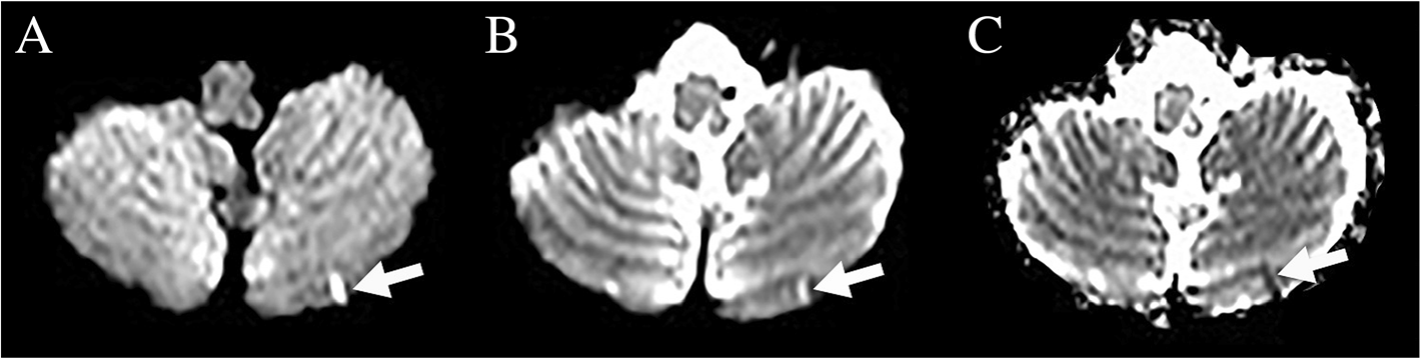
MRI showing a patient with acute SCCI, seen as hyperintensity on DWI B1000 (a) and B0 images (b) and as hypointensity on ADC map (c)
